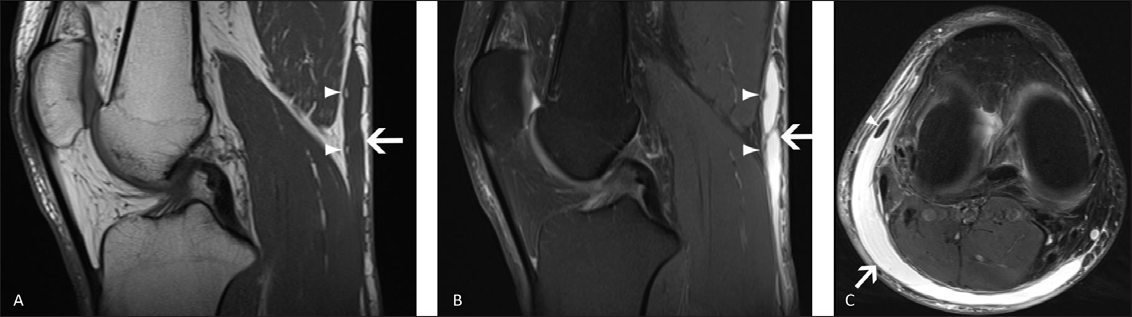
A 42-year-old man with a marked swelling of the lower leg after direct impact to the calf due to a motor vehicle accident. Type I lesion: seroma. Sagittal T1 (A), sagittal PD FS (B) and axial T2 (C) MR images demonstrate an oval smooth collection (white arrows) between the subcutaneous fat and the fascia of the calf muscle, demonstrating low SI on T1- and high SI on T2-WI. A small focal area of isointense signal compared to subcutaneous fat was seen within the lesion (white arrowheads), corresponding with an internal fat globule.
